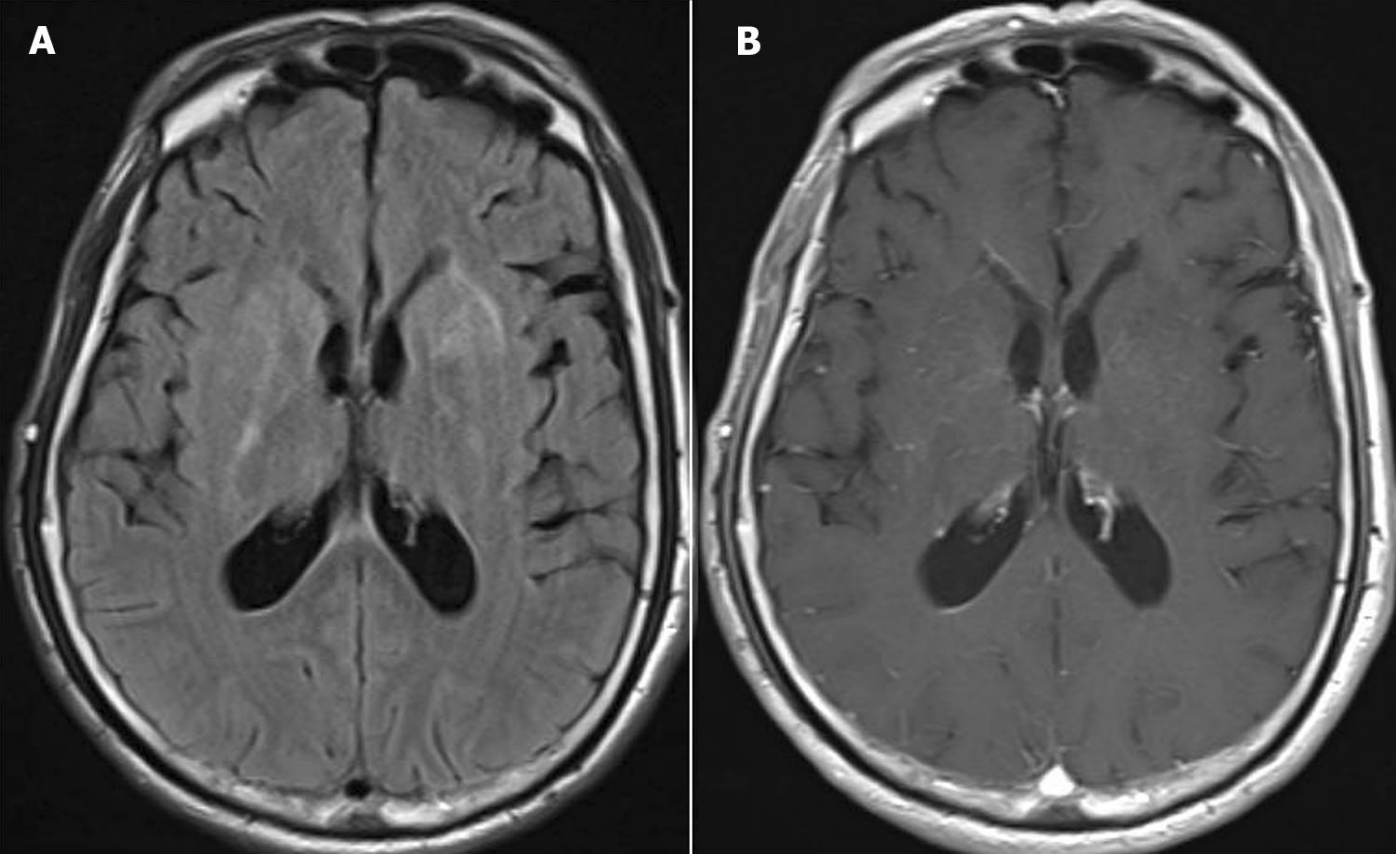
Brain MRI showing bilateral basal ganglia with the Flair image revealing high signals (A) without enhancement (B)
